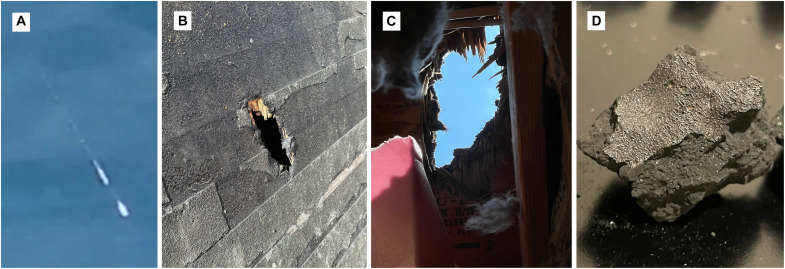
Impact and recovery of Hillsborough. (A) The daytime meteor with wake in an enhanced single video frame from Northford, CT. (B) The impact site in Hillsborough (roof tile width, 14 cm). (C) View of the impact site from inside. (D) Meteorite fragment with fusion crust. Photo credits: (A) M. Kirschner and M. Hankey, American Meteor Society; [(B) to (D)] A. Gordon.
